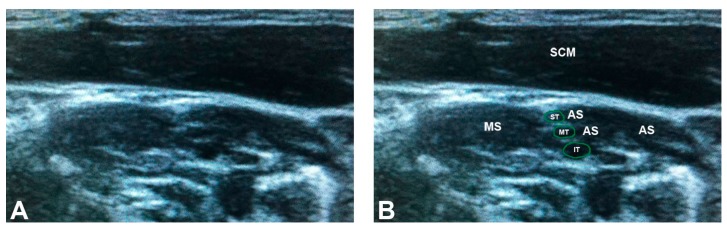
The multiple piercing variant, identified using ultrasonography in the present study: (A) unlabeled; and (B) labeled. Note that the superior and middle trunks are not separated from the anterior scalene in this condition, visible as a lack of hyperechoic fascia. AS = anterior scalene; C5 = anterior ramus of C5; C6 = anterior ramus of C6; IT = inferior trunk; MS = middle scalene; MT=middle trunk; SCM = sternocleidomastoid; ST= superior trunk. The green outlines demarcate the trunks and roots of the brachial plexus.
